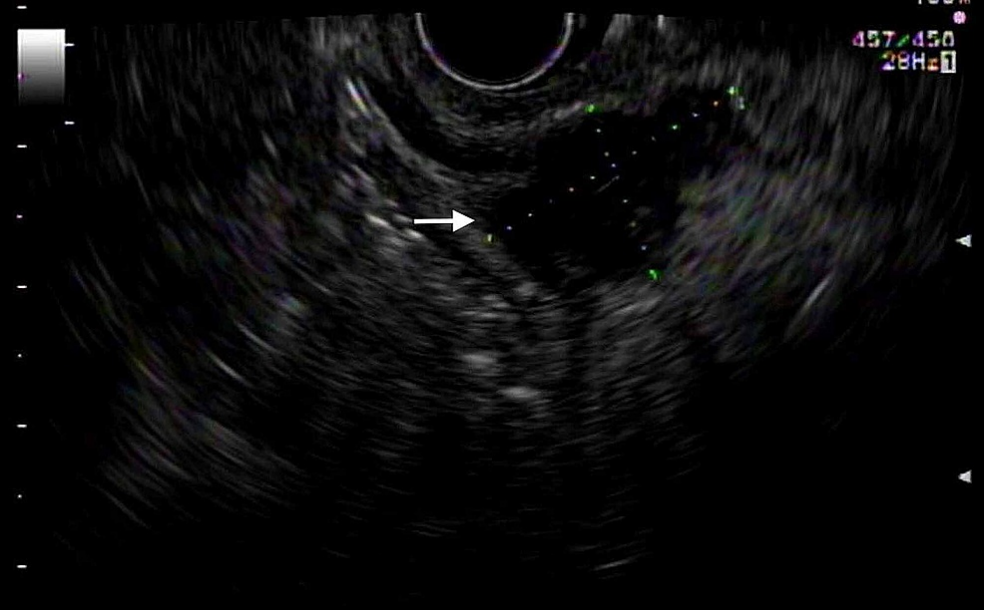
Endoscopic ultrasound showed a hypoechoic circumferential mass measuring 25 mm by 15 mm in maximal cross-sectional diameter; there was sonographic evidence suggesting invasion into the muscularis propria, as indicated by the white arrow.
